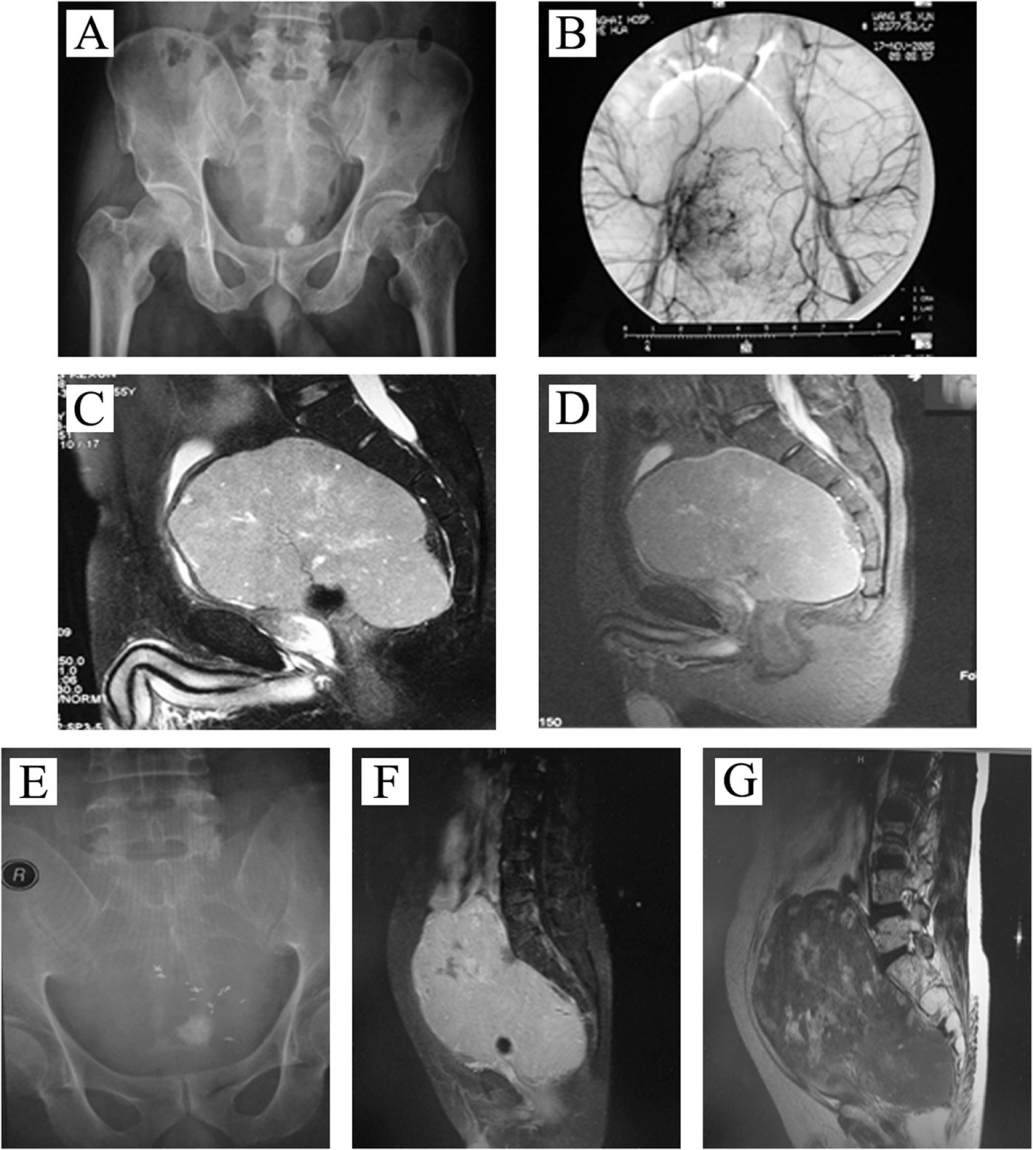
The X-ray films and computerized tomography (CT) scans of patient A before and after gelatin sponge particles (GSPs) combined with CT-guided ^125^I seed (*n* = 30) implantation treatment. a X-ray film of the pelvis taken in March 2005; b pelvic angiography showing the giant tumors before sacral in March 2005; c, d the CT scan taken in March 2005; e X-ray film of the pelvis taken in May 2009 after gelatin sponge particles combined with CT-guided ^125^I seed (*n* = 30) implantation; f the CT scan taken in October 2010; g the CT scan taken in September 2011
